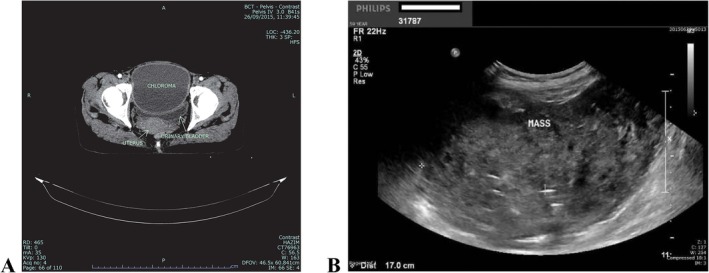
(A) CT and (B) ultrasound scan pictures of the pelvic mass.
